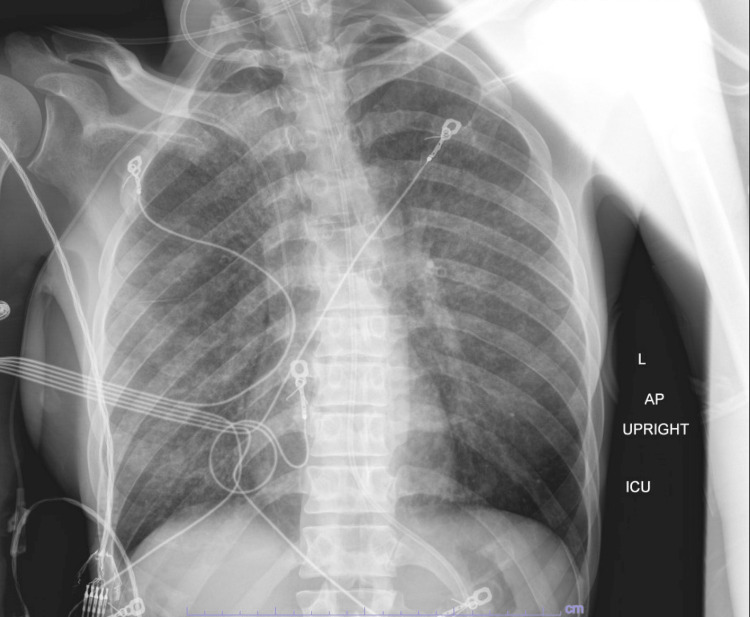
Chest X-ray at admission showing diffuse bilateral pulmonary infiltrate
